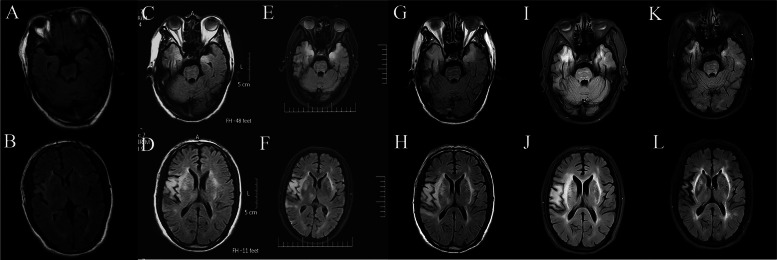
The plain brain MRI at different time after onset [(A.B) 9th day, (C.D) 48th day, (E.F) 54th day; (G.H) 69th day;(I.J)94 day; (K.L)154 day;] after initial symptom. (A.B) Brain MRI -FLAIR scans revealed hyperintense signal changes in the right frontal lobe, insula cortex. (C.D) New lesions appeared in the right temporal lobes, left hippocampus, bilateral insular lobe, brain stem and right basal ganglia at the 48th day after initial symptoms. (E.F) FLAIR analysis show compared with Figure C.D, there was also a new lesion in the right thalamus at the 54th day. (G.H) In contrast to Figure E.F, the lesions in the bilateral temporal and insula are enlarged at the 69th day. (I.J) There was no further enlargement of the lesion at the 94^th^ day.(K.L) On day 154 after the onset, the patient's symptoms improved, and follow-up MRI showed that all lesions were significantly reduced
